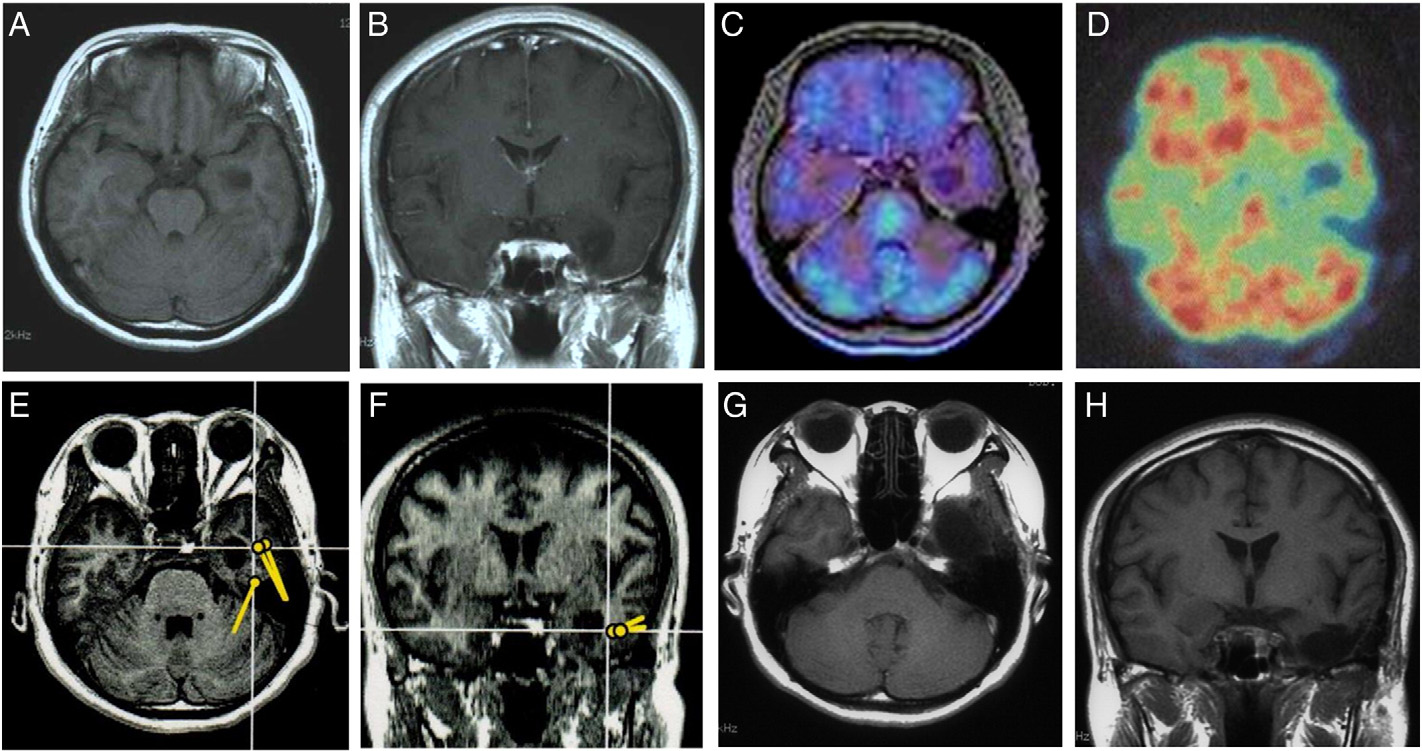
Case 1. T1WI showing a relatively well-demarcated, small hypointensity mass lesion in the left medial temporal lobe in axial (A) and coronal (B) images. The tumor shows low uptake in MET-PET (fused with T1WI) (C) and hypo-uptake in FDG-PET (D). Preoperative interictal MEG shows clustered dipoles in the region lateral to the tumor (E and F). Postoperative T1WI showed the tumor to be totally resected (G and H).
